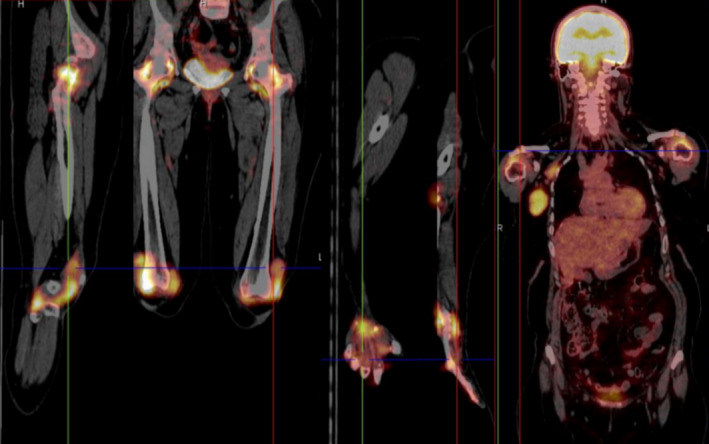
18FDG PET/CT image shows an abnormal uptake of FDG in DIP, PIP, wrists, shoulders, knees, right axillary lymph nodes and cutaneous lesions
